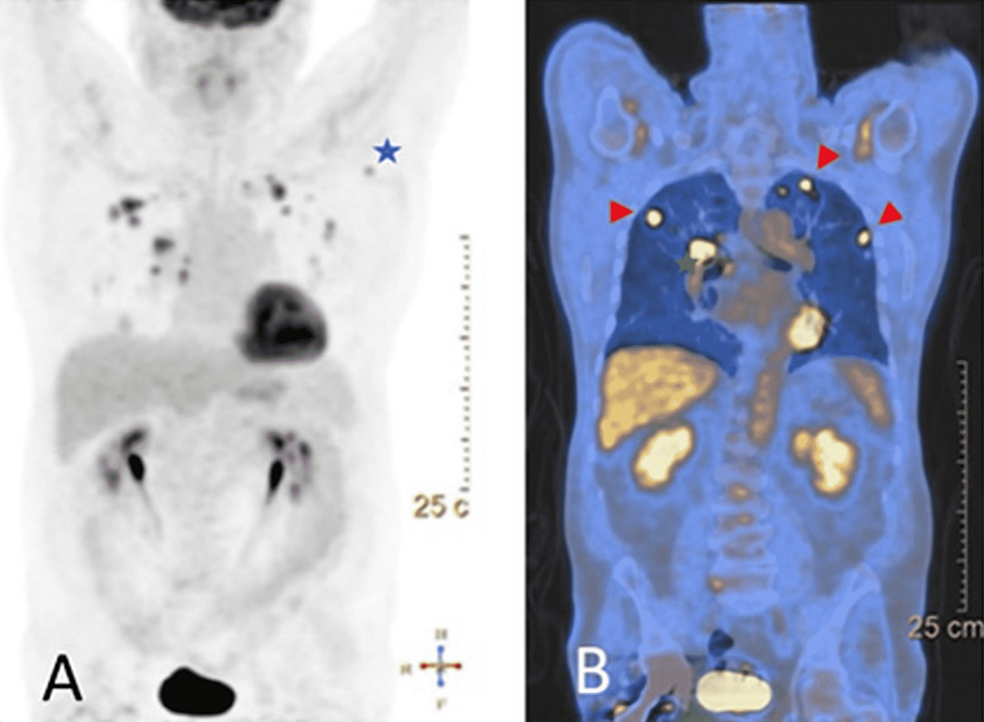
Initial positron emission tomography–computed tomography (PET/CT) findings. A) maximum intensity projection (MIP) (Blue star: hypermetabolic axillary lymphadenopathy). B) coronal-fused PET/CT demonstrates multiple bilateral hypermetabolic pulmonary nodules, intense fluorodeoxyglucose (FDG) avid right hilar metastatic lymphadenopathy, and metastatic hypermetabolic left axillary lymph node (Red arrowhead: hypermetabolic pulmonary nodules, Green star: hilar metastatic lymphadenopathy).
